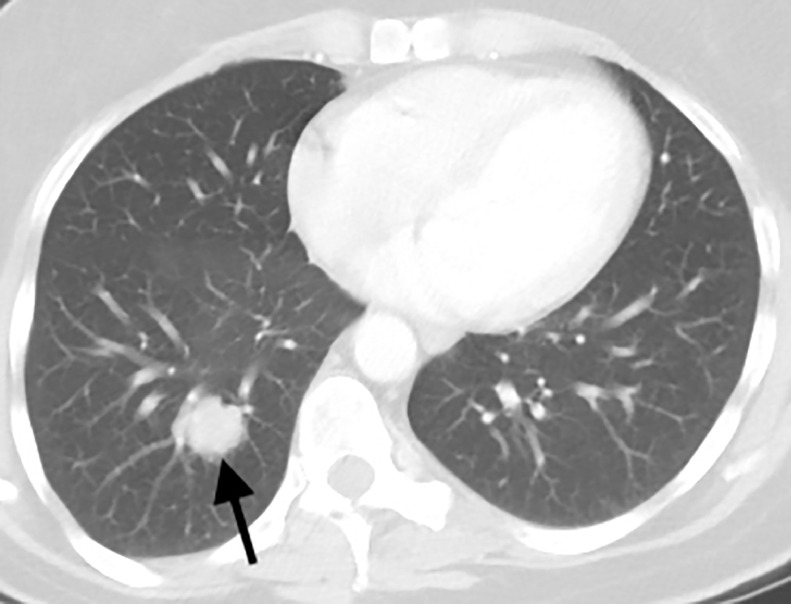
CT demonstrating a 2.2 cm right lower lobe pulmonary nodule abutting the bronchovascular bundle (arrow).
